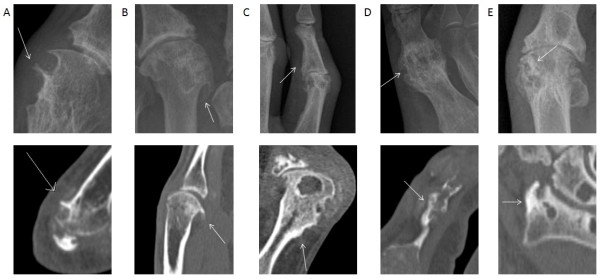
Examples of the features of new bone formation (NBF) observed by plain radiography and computed tomography in patients with gout. A. Spur. B. Osteophyte. C. Periosteal NBF. D. Ankylosis. E. Sclerosis. Top panel shows plain radiographic images and lower panel shows CT images. CT, computed tomography.
